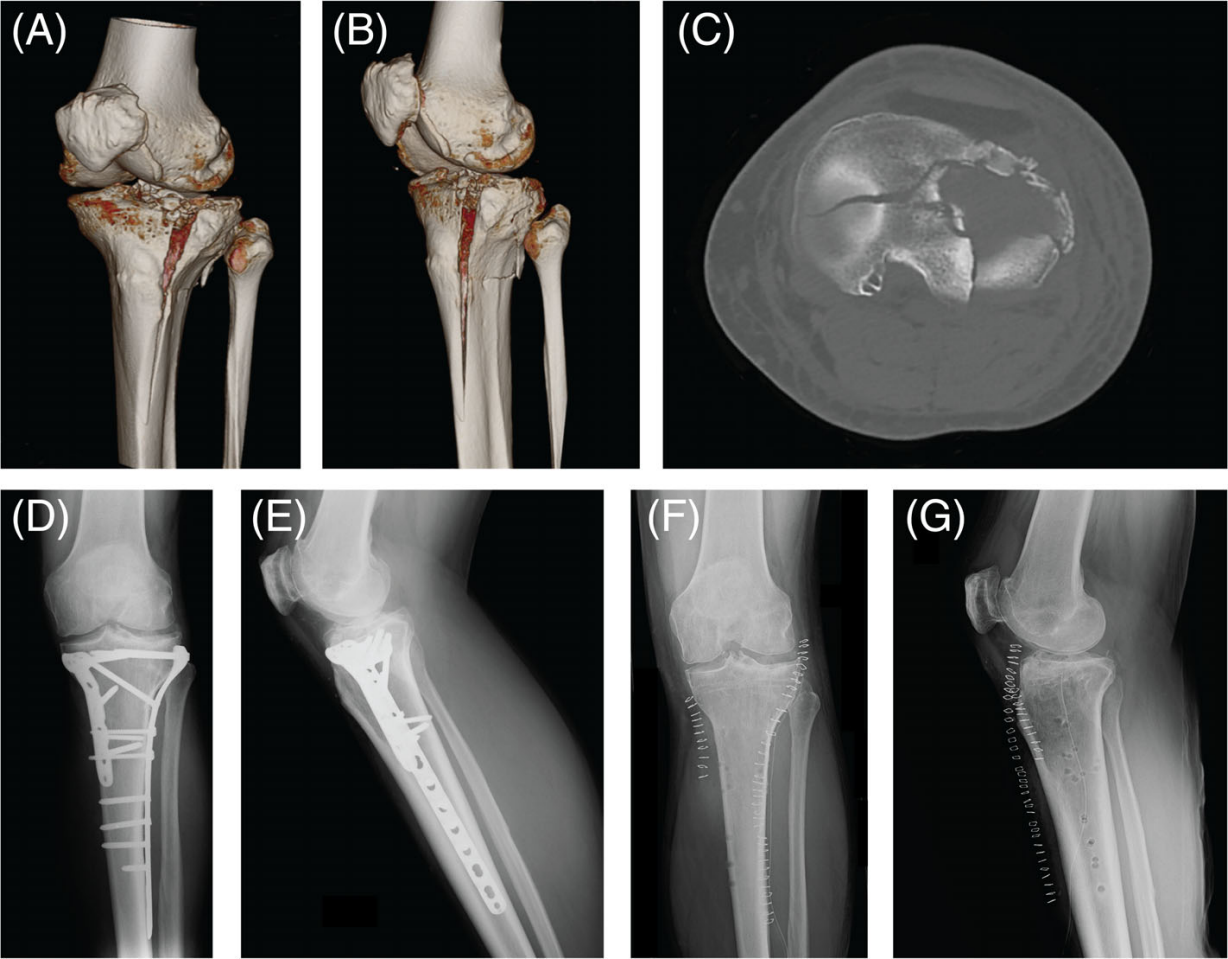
Case 1: A 56‐year‐old man with an AO 41‐C3 fracture treated by open reduction internal fixation (ORIF) technique. (A, B) Preoperative 3D‐CT scan reconstruction images; (C) CT axial image; (D, E) Anteroposterior and lateral radiographic images 6 months after surgery; (F, G) Anteroposterior and lateral radiographic images after removal of implants 35 months after surgery.
